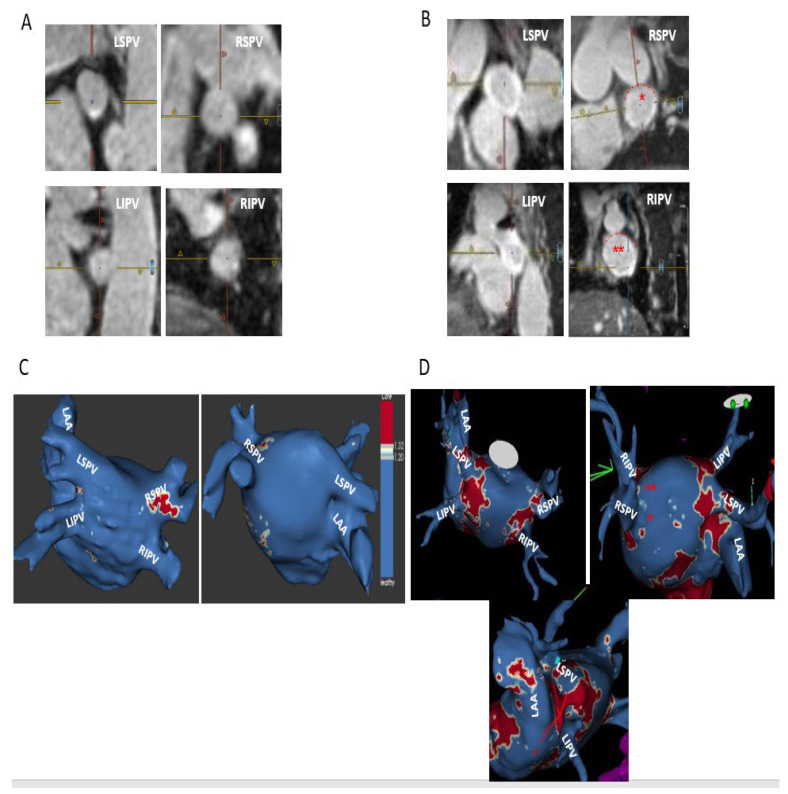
A typical patient with cryoballoon-induced partial incomplete circumferential fibrosis in both right PVs. Shown are pre-ablation (A) and post-ablation (B) cross-sectional short-axis views of the four PVs revealing minimal PV ostial fibrosis at baseline, while at post AF ablation, there is a complete circumferential fibrosis around both left PVs and partial incomplete circumferential fibrosis around both right PVs with major gaps in the roof and anterior segments of both veins (red dotted lines). (C,D) Corresponding ADAS 3D LGE model images pre (C) and post (D) cryoballoon AF ablation, showing minimal fibrosis at baseline (C). Post-ablation MRI (D) reveals a complete circumferential fibrosis around both left PVs (including the carinal view) and partial circumferential fibrosis of both right PVs due to major gaps in the anterior and roof segments of both RSPV (*) and RIPV (), corresponding and matching with the gaps seen on the “raw data” short-axis views. Post-ablation fibrotic areas on ADAS are colored red. Notably, Figure 1 and Figure 3 both describe the same patient.
